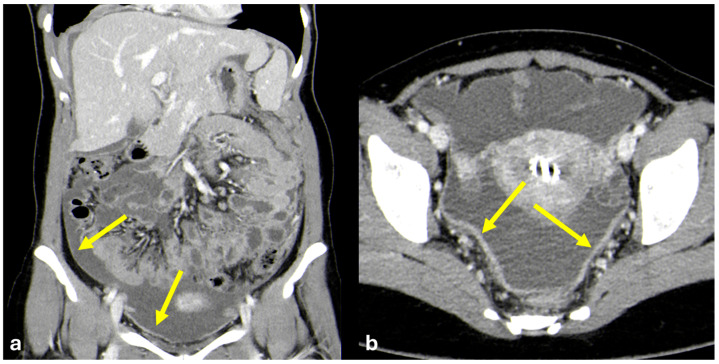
Tubercular peritonitis. A young woman with progressive abdominal pain and an increased abdominal circumference. Portal venous phase abdominal CT is performed due to the suspicion of oncological disease and shows abundant peritoneal fluid associated with diffuse and smooth thickening of the parietal peritoneum ((a): coronal and (b): axial, arrows) with no focal thickening or nodules.
